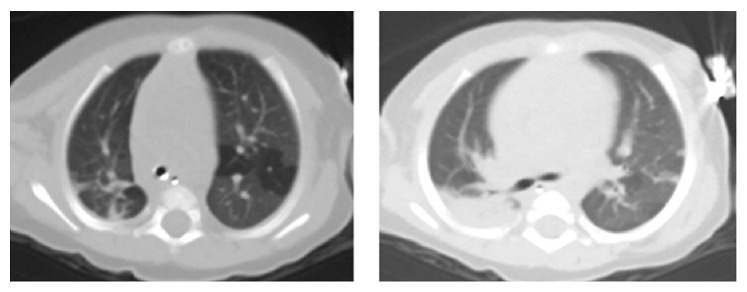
CT chest: bibasilar atelectasis with several areas of air trapping bilaterally. No definite evidence of interstitial lung disease. Enlarged heart.
